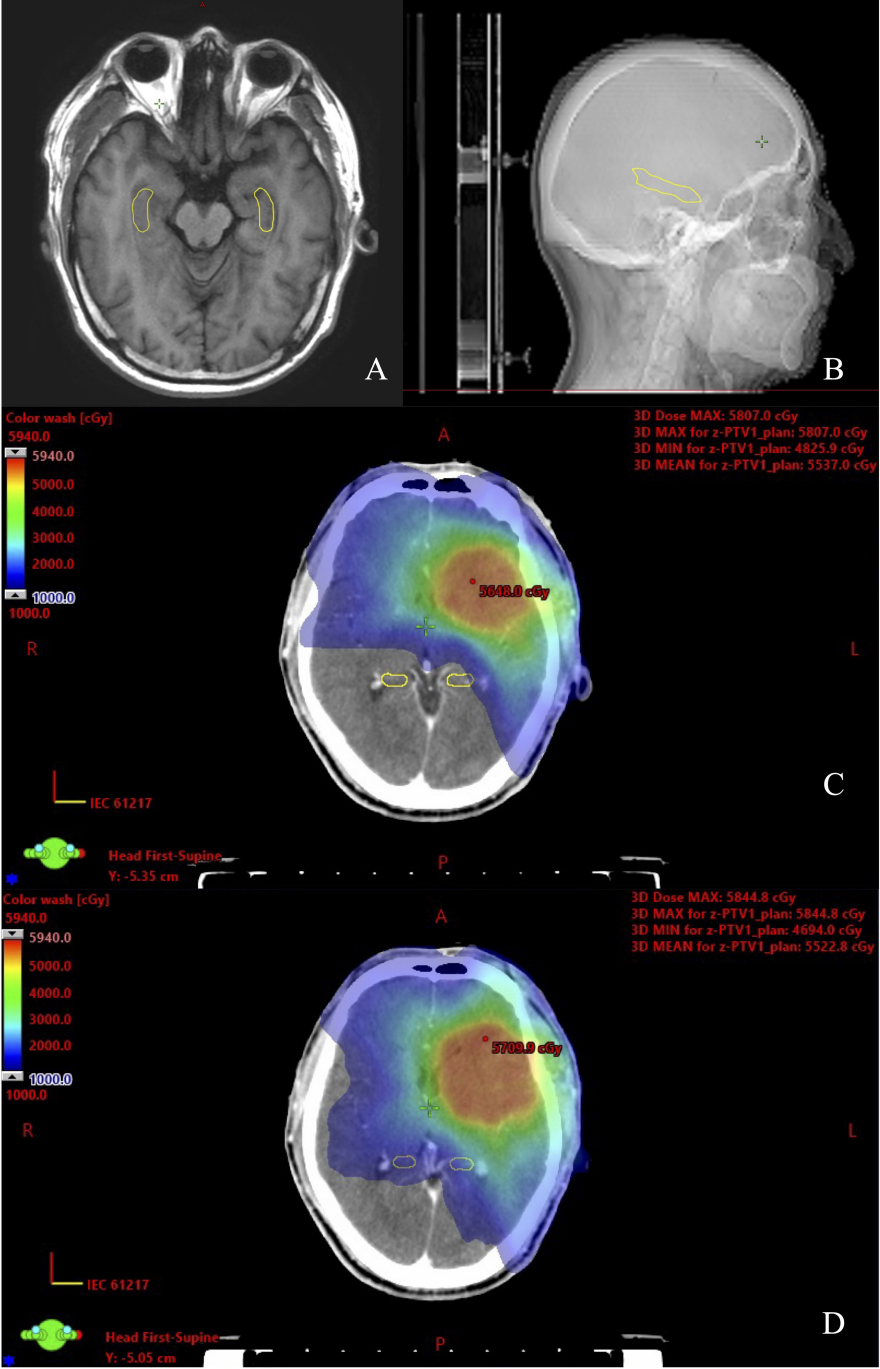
View of dose distribution of the hippocampus contoured on CT-MRI fusion for one glioma case. (A) Hippocampal contour in transverse plane. (B) Hippocampal contour in sagittal plane. (C) HS VMAT plans. (D) NHS VMAT plans.
